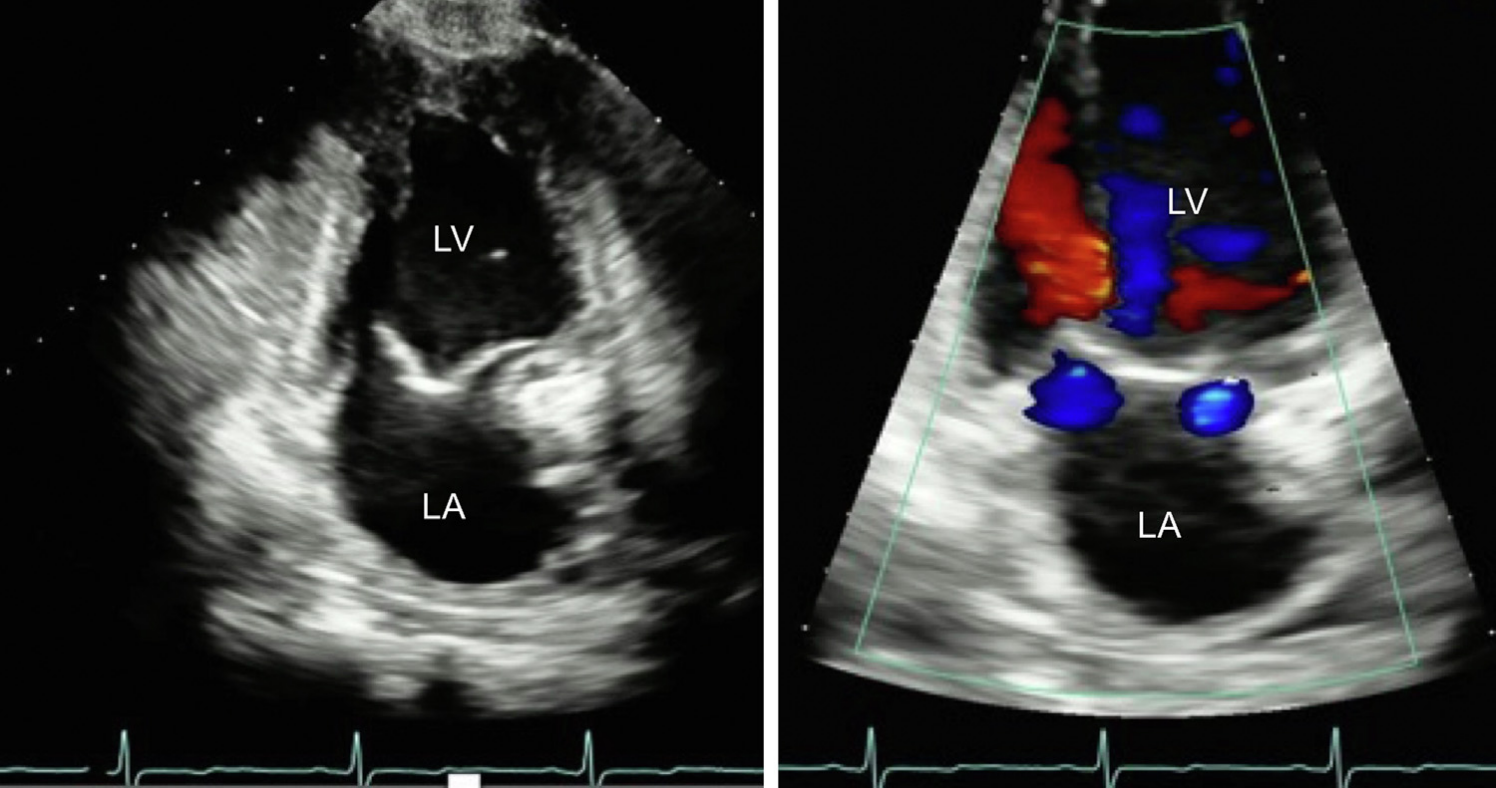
Two dimensional TTE, apical 2-chamber view, early systolic phase, without (*left*) and with (*right*) color flow Doppler demonstrates normal LV size and function with mild mitral regurgitation in both orifices. *LA*, Left atrium; *LV*, left ventricle.
